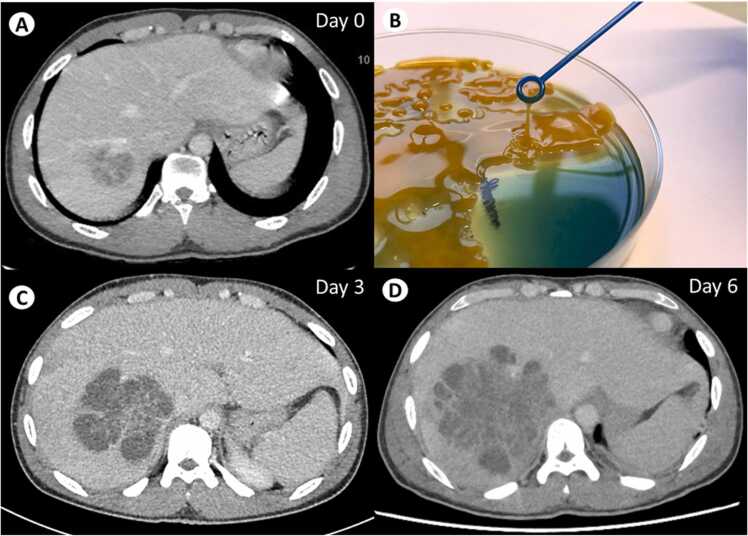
Abdominal computed tomography scan at the admission of the patient (day 0) showing a 40 mm liver abscess (A). Positive string test of the hypermucous *Klebsiella pneumoniae* strain recovered from blood cultures (B). Abdominal computed tomography scan performed at day 3 (C) and 6 (D) following clinical deterioration and showing a progression in size of the multicompartmental liver lesion.
